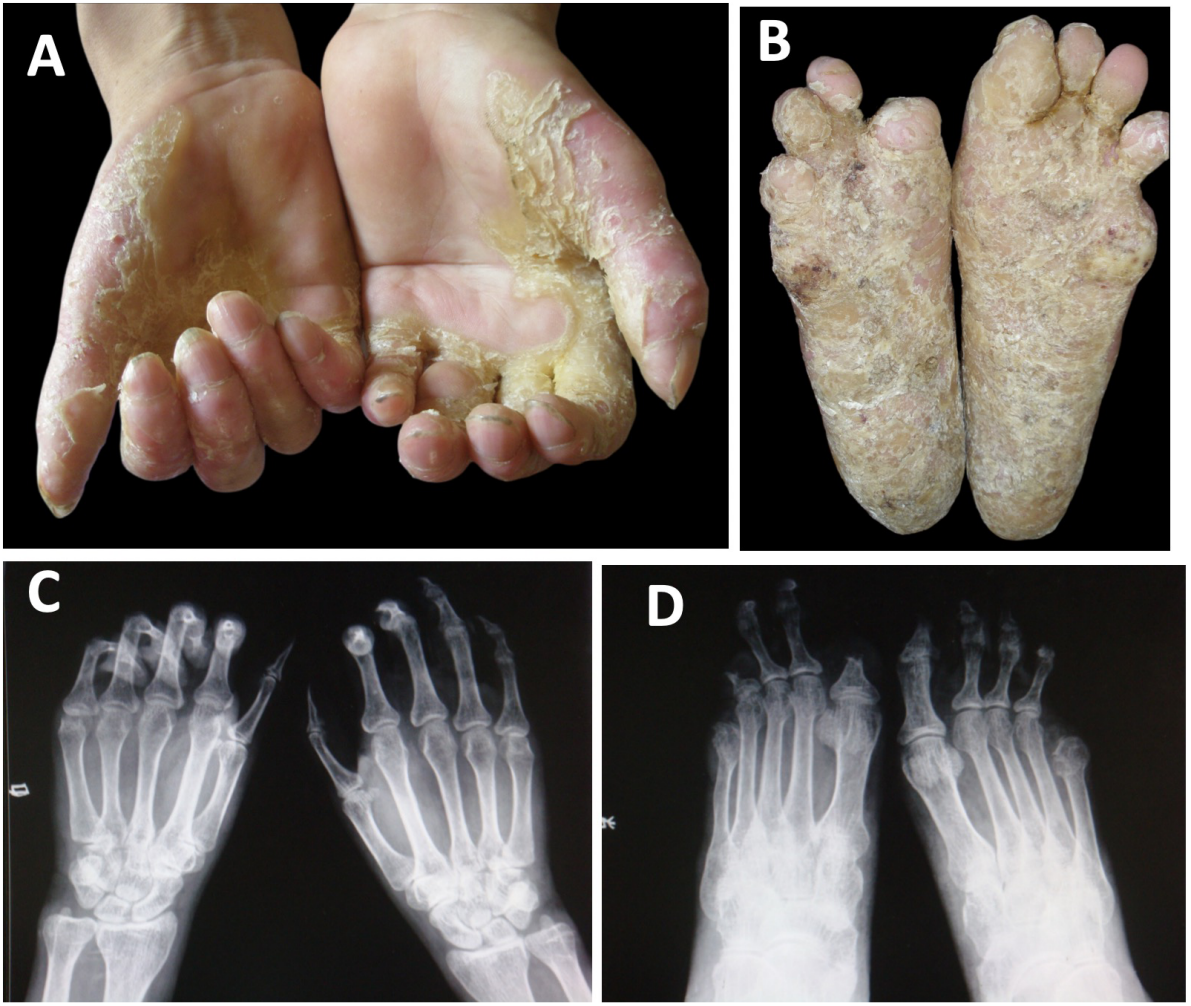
Clinical features of the patient. (A, B) Bilateral, mutilating palmoplantar keratoderma, flexion deformities, and constriction of digits were indicated. (C, D) The X-ray examination presented osteoporosis in the bones of both hands and feet, bending and deformation of phalanges. The destruction of some phalanges disappeared, and the joints were subluxated.
